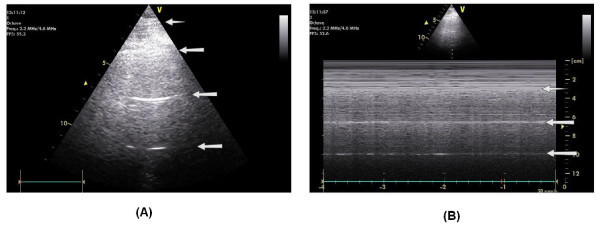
Normal lung ultrasound. (A) The pleural line (arrow): the A lines or horizontal lines arising from the pleural line are separated by regular intervals that are equal to the distance between the skin and the pleural line. (B) M mode shows the pleural line. Under the pleural line is the seashore sign (sandy pattern) due to the lung dynamics and pleural sliding. The horizontal lines are A lines, separated by regular interval (arrows).
